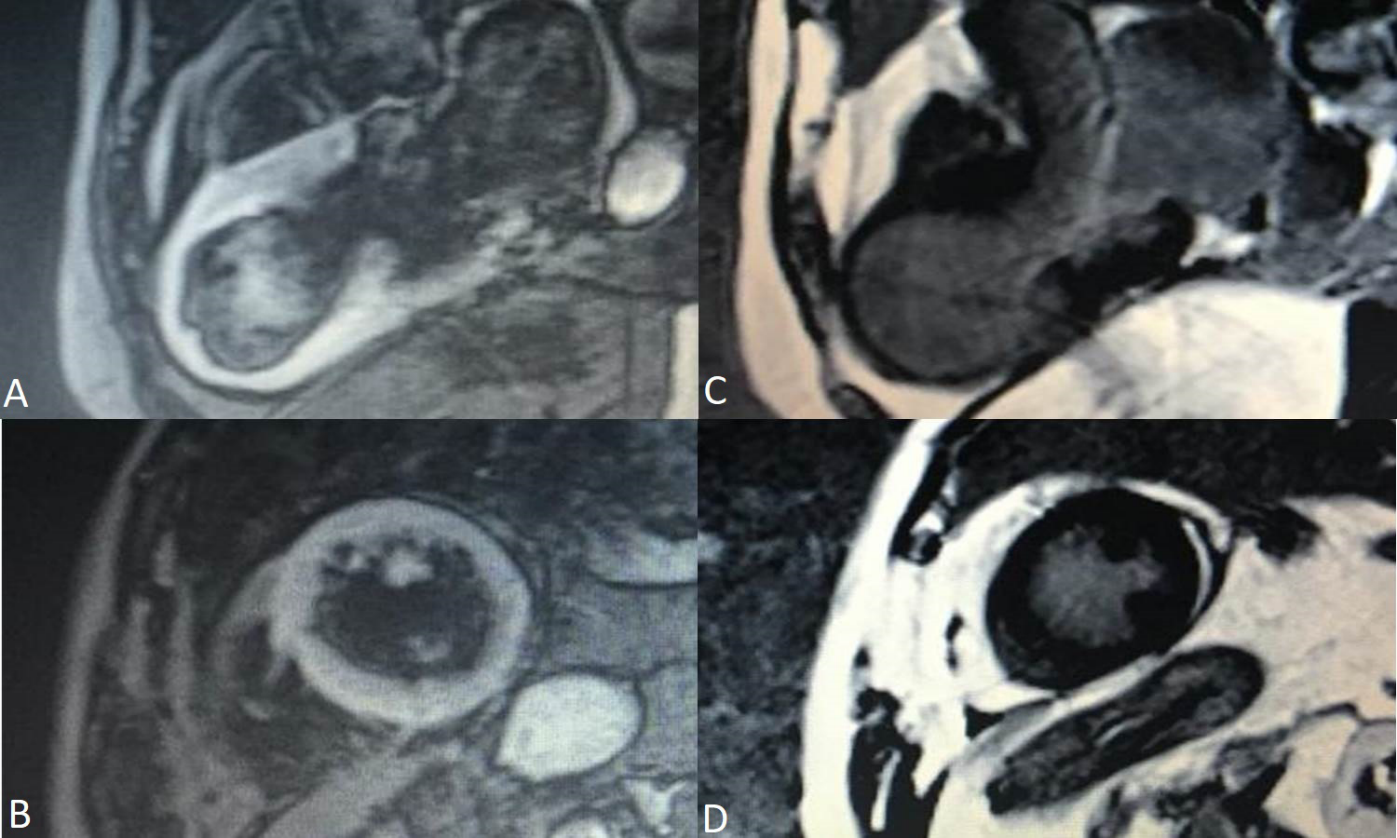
Cardiac Magnetic Resonance Imaging of a 78 years old female
